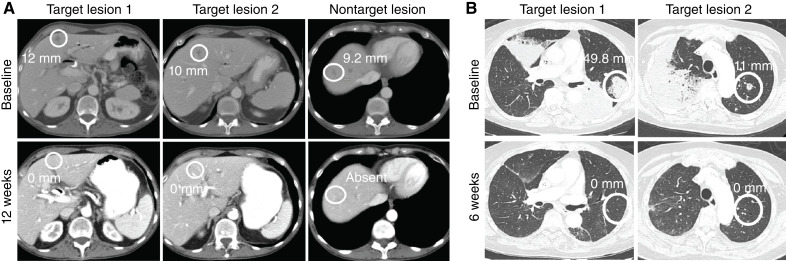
Activity of RMC-6236 in pancreatic and lung cancer patients. A, Pretreatment and 12-week (post cycle 4) scans of a heavily pretreated patient with a *KRAS*^G12D^ mutation-positive PDAC indicating a complete response of both target and nontarget lesions. Patient continued on study treatment in cycle 6. Axial views of computed tomography (CT) abdomen images prior to RMC-6236 treatment (top) and after four cycles of RMC-6236 treatment (bottom). B, Pretreatment and 6-week (post cycle 2) scans of a patient with a *KRAS*^G12V^ mutation-positive NSCLC indicating a complete response of target lesions (no nontarget lesions present at baseline), atelectatic changes in the right lung are also largely resolved by 6 weeks. Complete response was confirmed at cycle 4, and the patient continued on study treatment with a complete response in cycle 10. Axial views of computed tomography (CT) chest images prior to RMC-6236 treatment (top) and after two cycles of RMC-6236 treatment (bottom).
